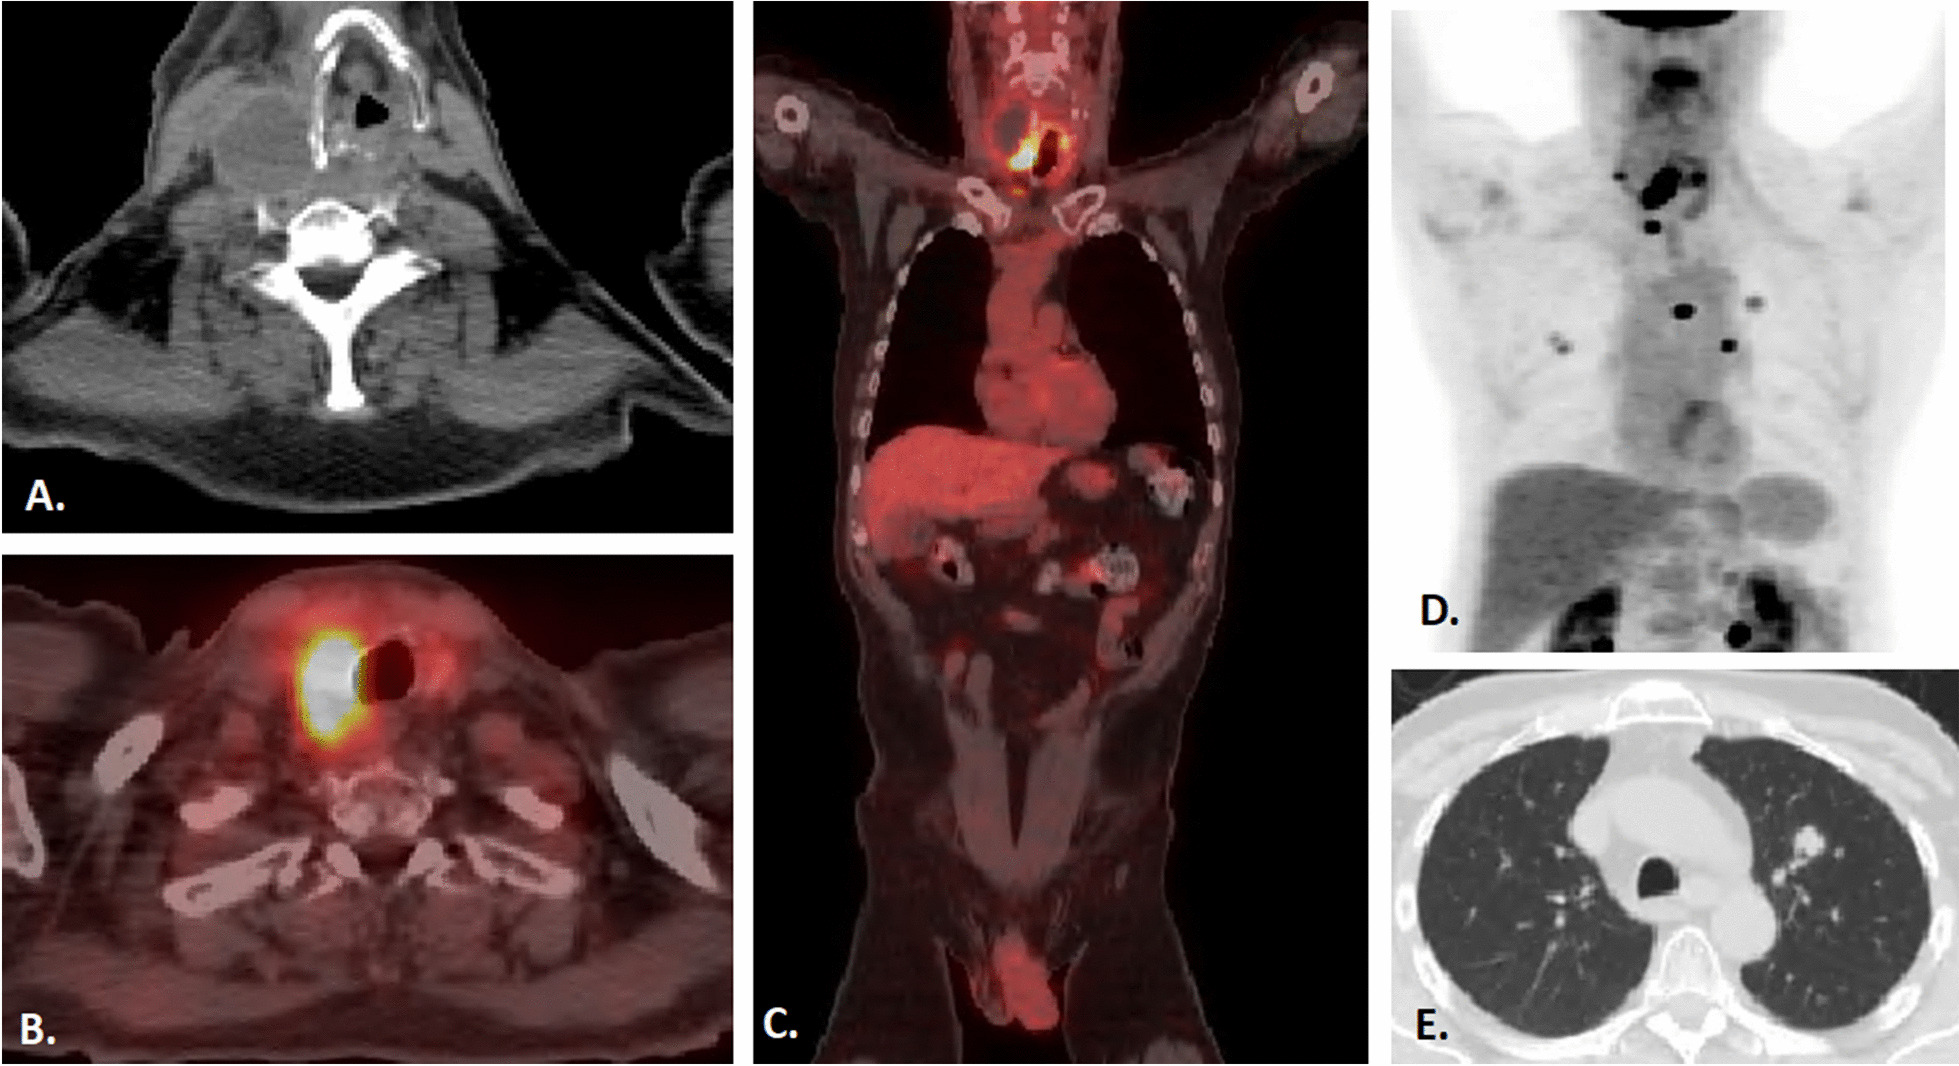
Post-concurrent chemoradiation PET/CT imaging with carboplatin/paclitaxel reveal a smaller thyroid tumor size (A) and decreased PET-avidity (B, C), but show new bilateral pulmonary metastases (D, E)
